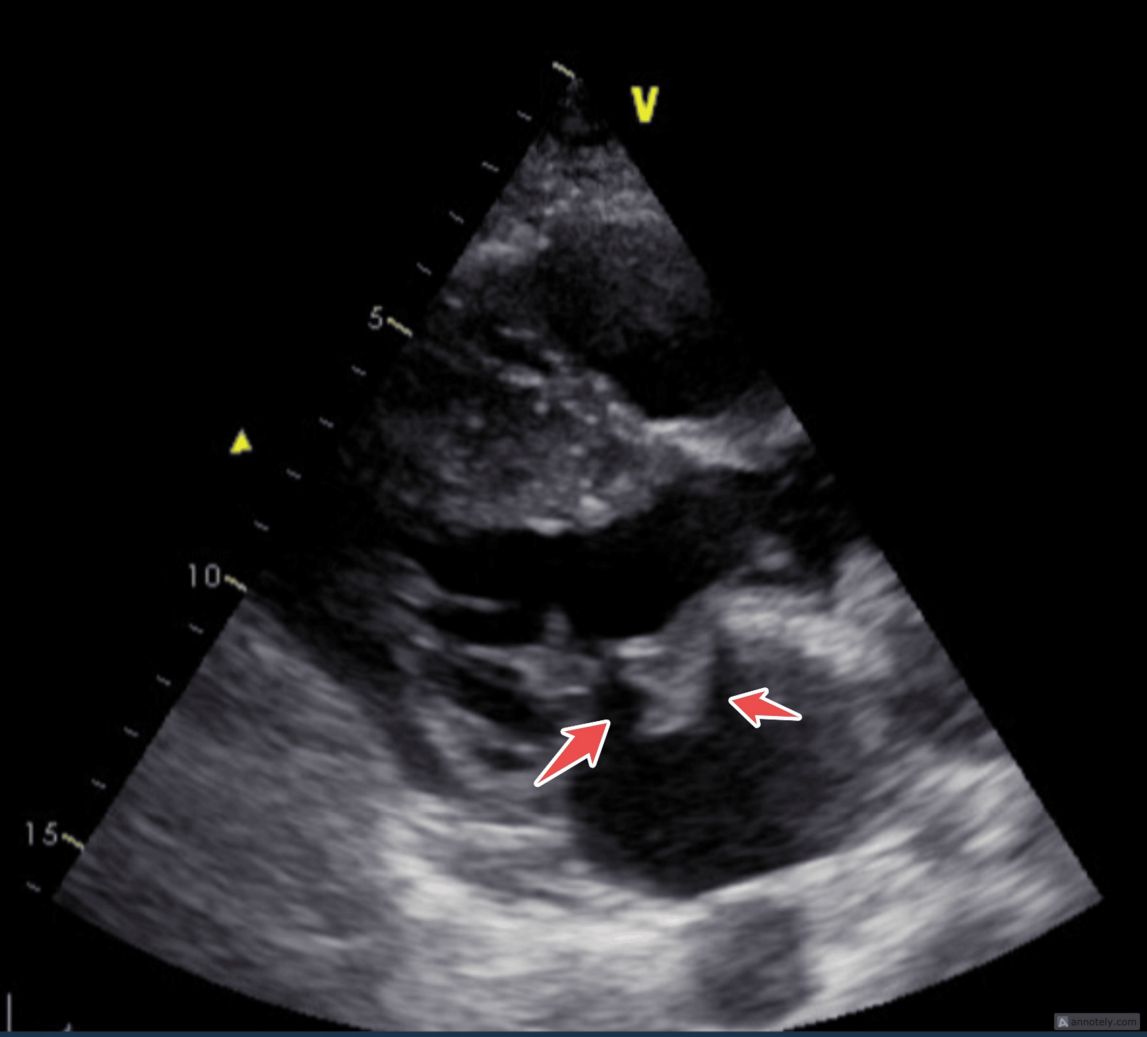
PLAX view showing mitral valve prolapse with vegetation, highlighted by arrows. PLAX: Parasternal Long Axis.
